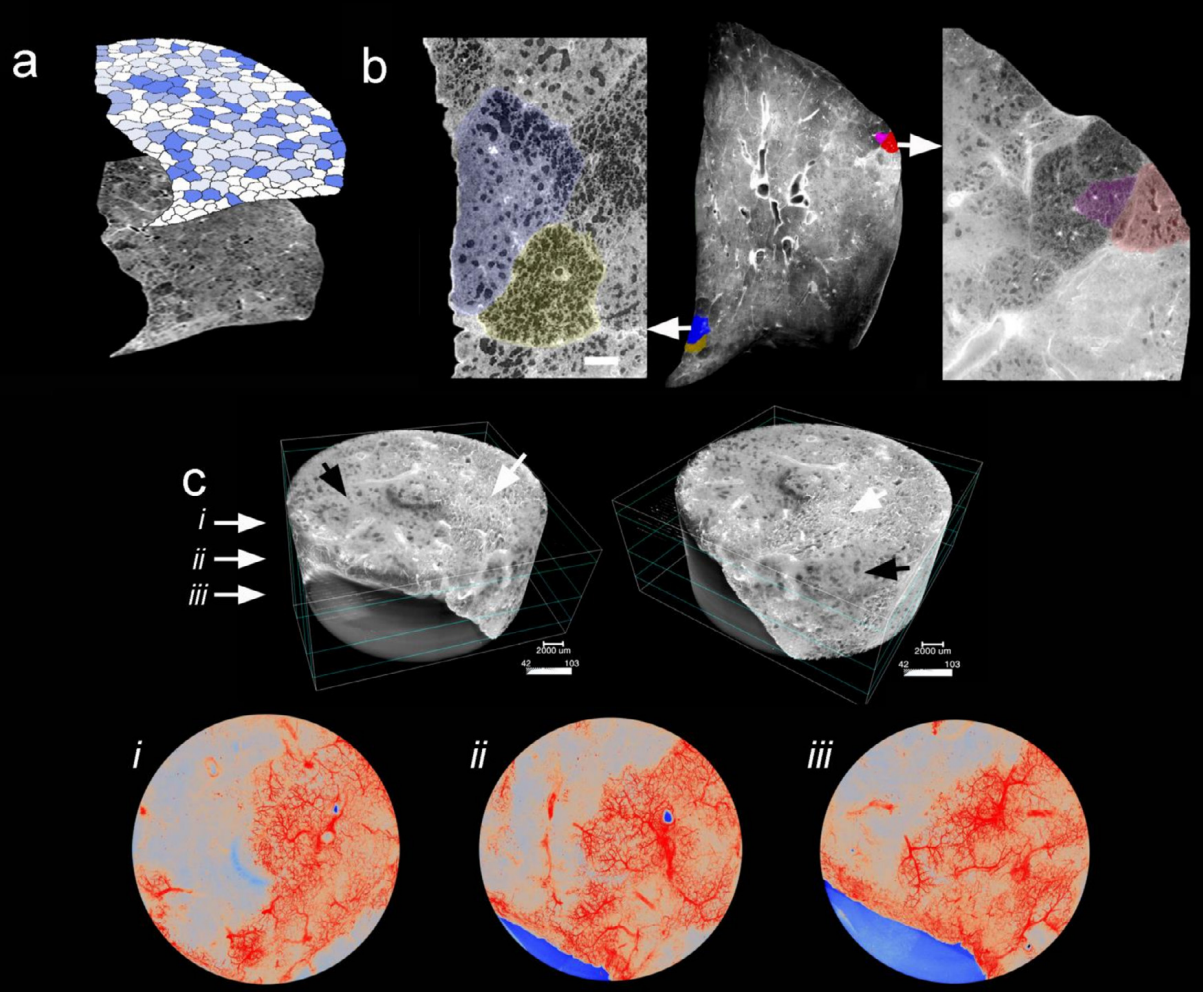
Synchrotron imaging of COVID-19 autopsy lungs. (a) Hierarchical phase-contrast tomography (HiP-CT) reconstructions segmented for secondary pulmonary lobules involved with microischemia. (b) Segmentation of individual secondary pulmonary lobules via HiP-CT depicts the presence of severely abnormal-appearing secondary lobules immediately juxtaposed to nearly normal-appearing lobules. (c) HiP-CT demonstrates the dramatic paucity of blood flow in diseased secondary lobules in the vicinity of fibrotic remodelled areas.
